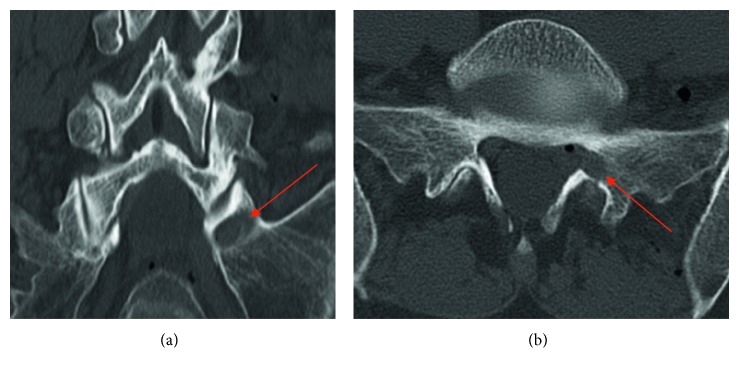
Lumbar CT 1 day after surgery of case 3 with bone hole of the pedicle.
